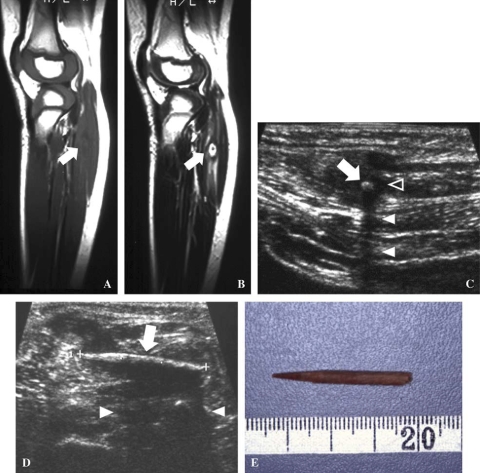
Three-year-old girl with foreign body granuloma of the right posterior lower leg. A and B: Sagittal T1- and T2-weighted images of the right posterior lower leg. The mass had low intensities on T1- and high intensities on T2-weighted images (arrows). Central area of the lesion had low intensities both on T1- and T2-weighted images. C and D: Sonography imaging perpendicular and horizontal to the foreign body. The foreign body was observed as a hyperechoic lesion (arrow) with posterior acoustic shadowing (solid arrowheads) and hypoechoic halo (open arrowhead). E: A 3-cm long tip of toothpick was removed at surgery.
